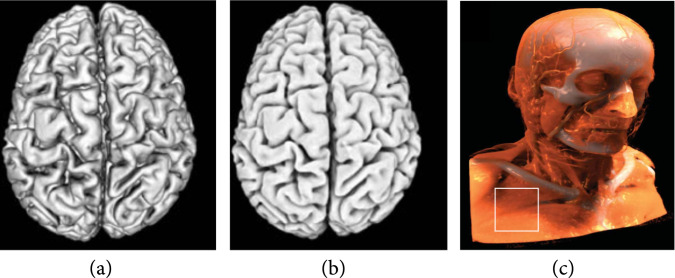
Direct volume rendering of a brain scan using (a) the traditional local illumination model and with (b) the directional occlusion shading [35] that approximates global illumination to enhance depth perception. ©2009 John Wiley & Sons, Inc. Reprinted, with permission, from Schott et al. [35]. A more advanced global illumination technique [36] generates the photorealistic rendering of a CT scan in (c). ©2014 IEEE. Reprinted, with permission, from Ament et al. [36].
